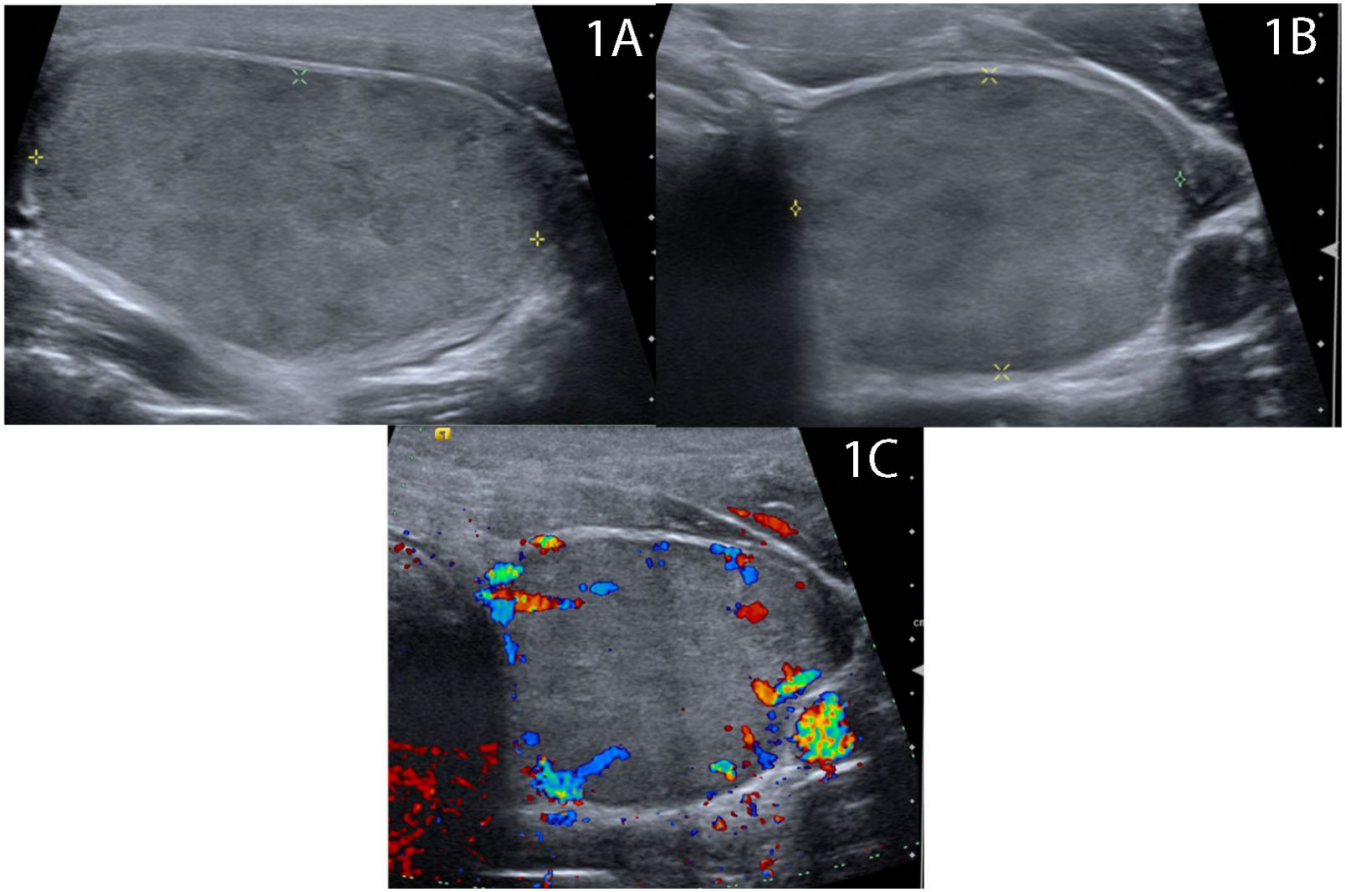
Baseline ultrasound demonstrates 4.2 cm solid homogenous, mildly hypoechoic nodule thyroid nodule replacing the majority of the left thyroid lobe in (A) longitude and (B) transverse views with (C) scant peripheral vascularity.
